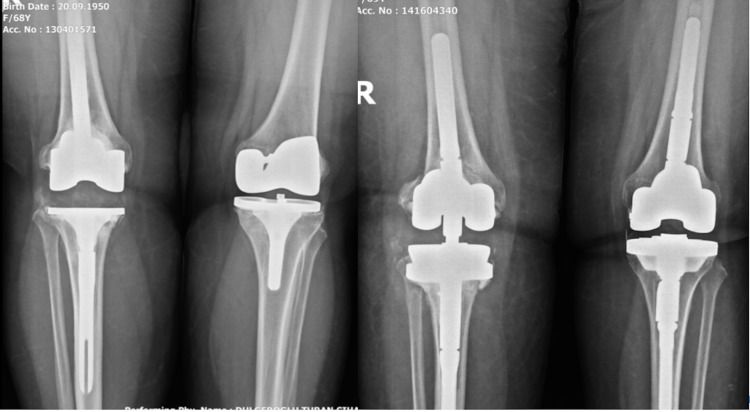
Case 4 Preoperative and Postoperative Knee X-Ray
